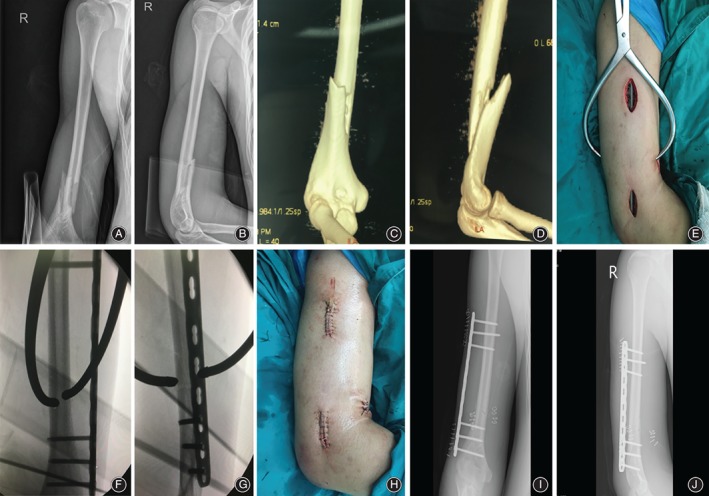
Preoperative anteroposterior (A) and lateral (B) X‐ray films of a distal‐third diaphyseal humerus fracture. The preoperative CT three‐dimensional reconstruction (C, D) shows obvious fracture displacement. During the surgery, the forceps were used to assist the fracture reduction through two small percutaneous incisions at anterior and posterior, and LCP was inserted lateral subcutaneously through two small incisions (E). Anteroposterior (F) and lateral (G) intraoperative fluoroscopy after fixation shows that the fracture achieved good reduction and the LCP was unattached to the bone surface. The incisions after suture were small (H). Anteroposterior (I) and lateral (J) X‐ray films 3 days after surgery shows that the fracture was in good position and firmly fixed.
